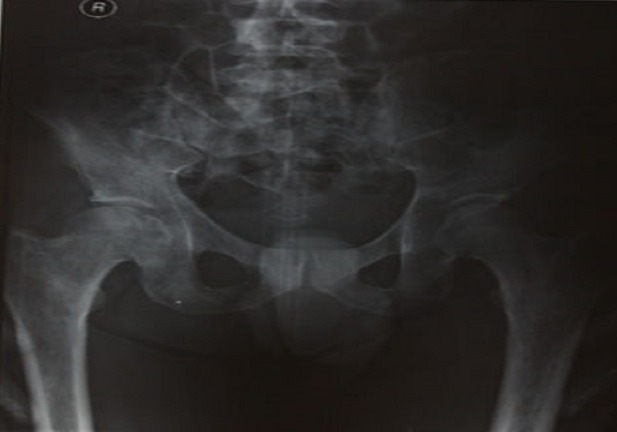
X-ray AP pelvis showing lytic bone lesions in the right femur
